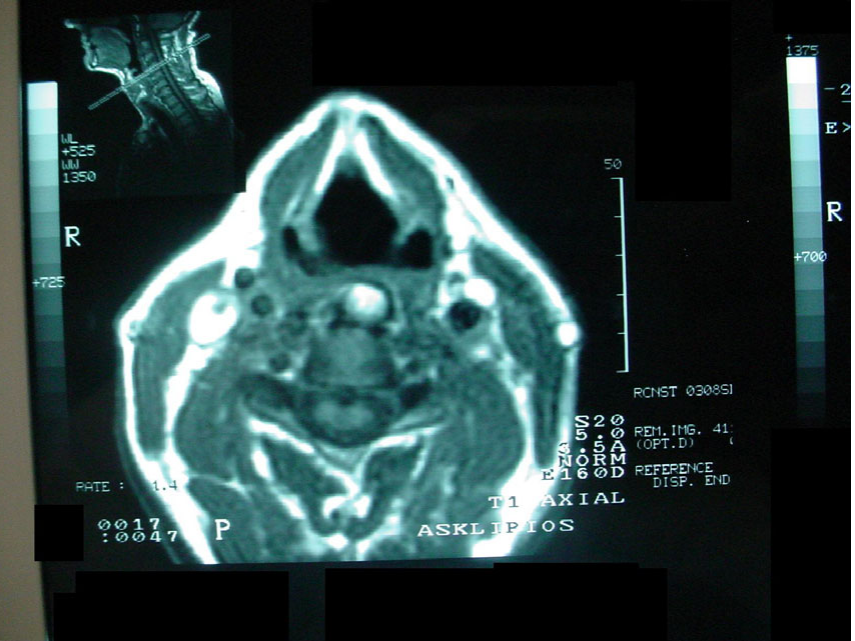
Sagittal MRI of the cervical spine showing the abnormal prevertebral tissue formatted by the osteophytes.
